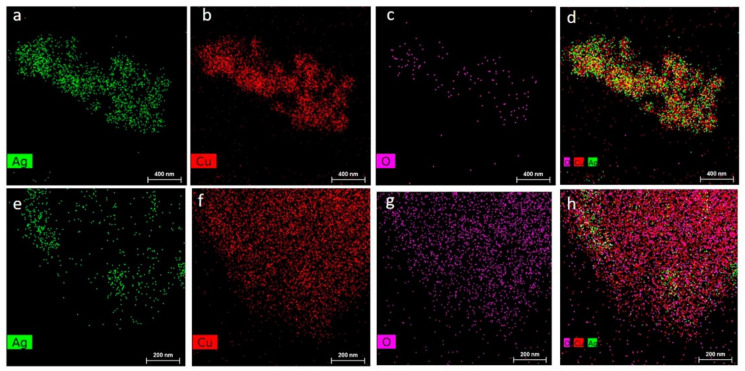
Energy-dispersive X-ray mapping for corresponding elemental distribution of Ag, Cu, and O on Ag_2_Cu_1_ (a–d) and AgCuO*_x_* NPs (e–h). Reprinted from [89] with permission from ACS Publications.
